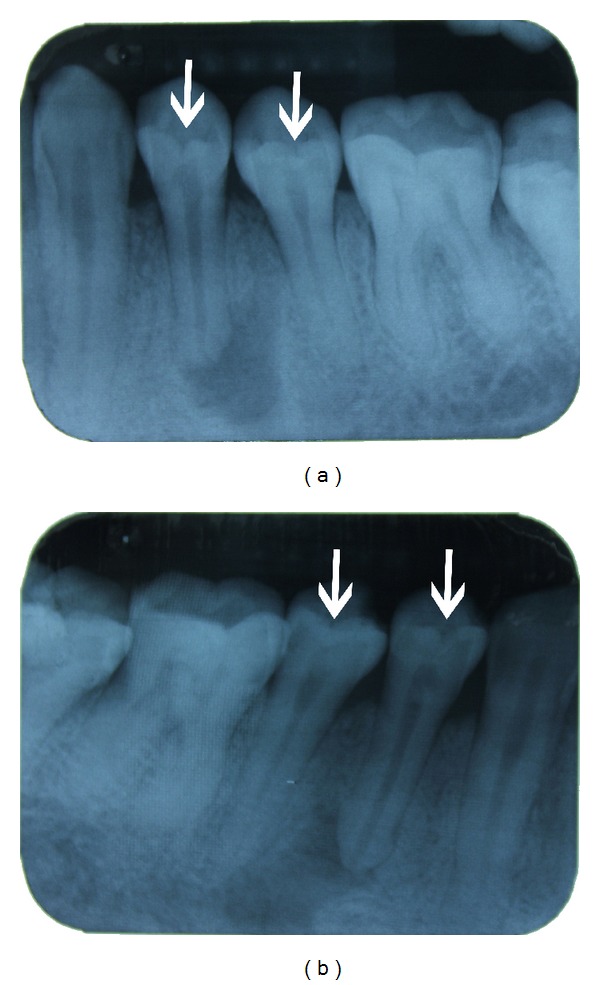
Long-cone periapical radiographs showing the left 1(a) and right 1(b) mandibular premolars. Well-demarcated periapical radiolucencies can be seen associated with the first premolar teeth bilaterally. The white arrows indicate radiolucent “invaginations” on the occlusal surfaces of the teeth.
